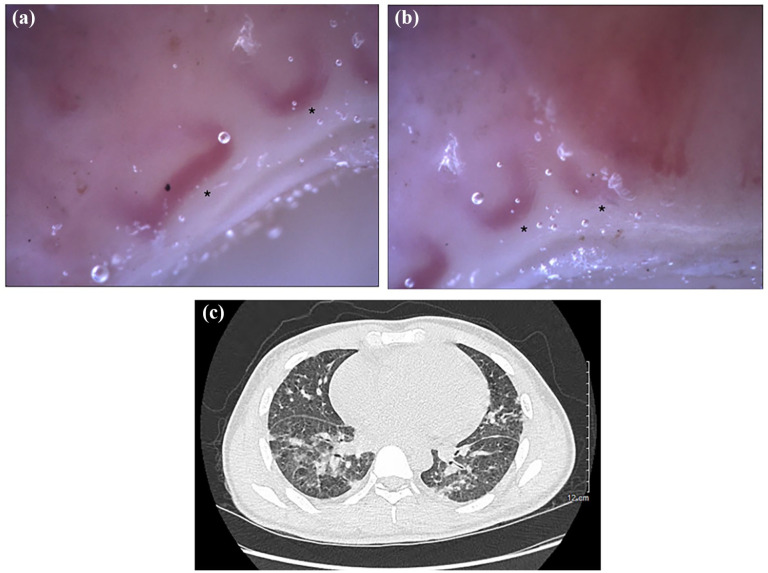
Case 1. (a, b) Panels show the patient’s nailfold videocapillaroscopic pictures at 200× magnification. (a) Panel shows markedly reduced capillary density (one capillary/1 linear mm) and giant capillaries (semi-quantitative score 3). (b) Panel also shows markedly reduced density (two capillaries/1 linear mm) and giant capillaries (semi-quantitative score 3). (c) Panel shows the patient’s CT chest with evidence of interstitial lung disease. *Giant capillaries. CT: computed tomography.
